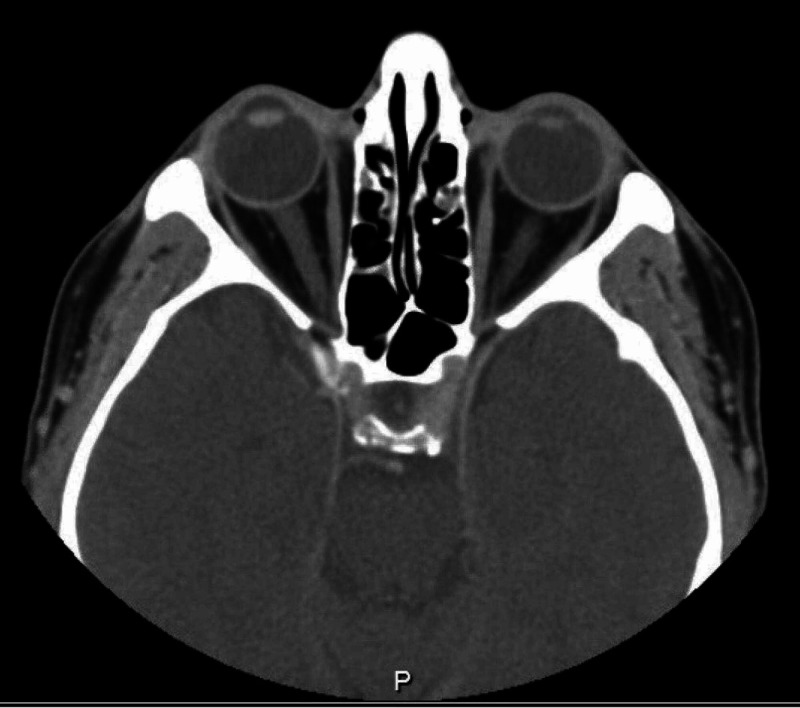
Brain and orbit computed tomography scan showing no abnormality in the orbital regions
